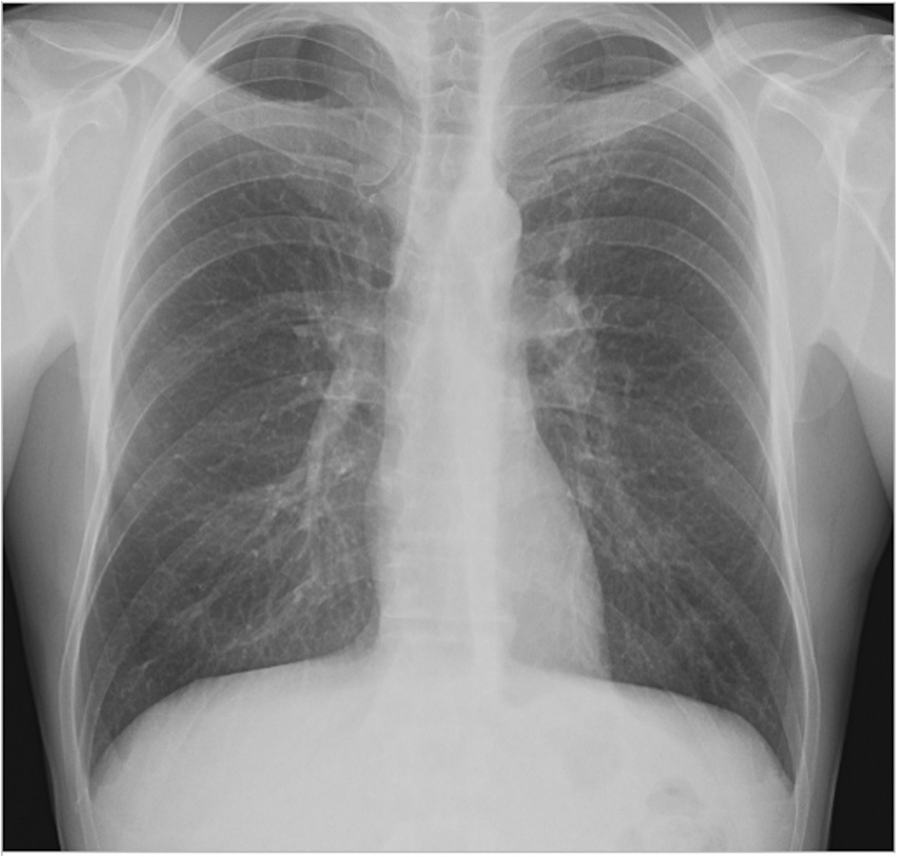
Follow up (post-operative) chest radiograph (PA view) of the patient showing a normal lung parenchyma of the left apex.
